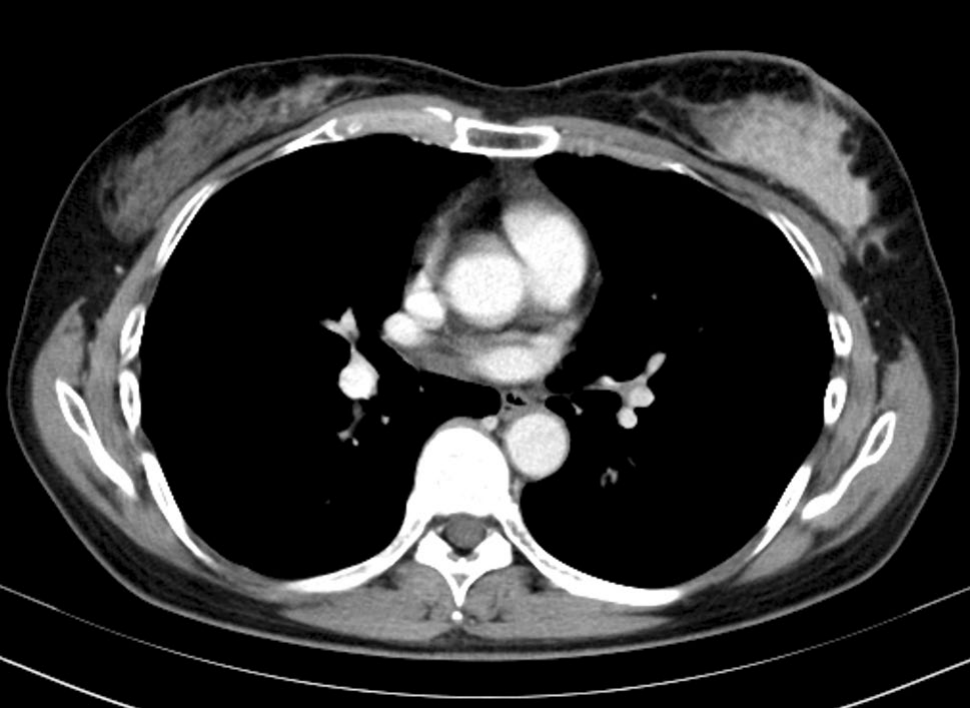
CT scan revealed a mass lesion measuring 62 mm in size in the left breast
